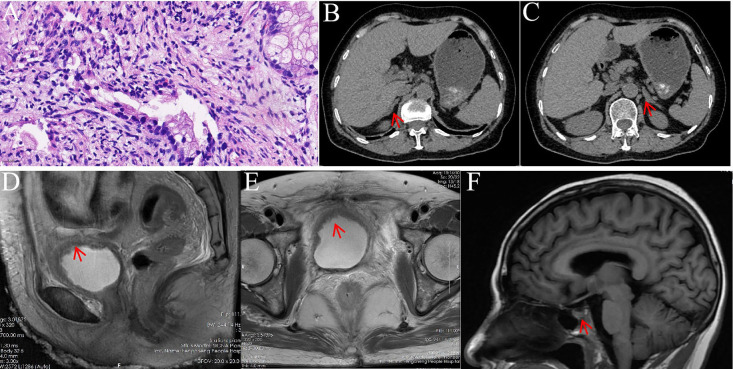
Pathological and imaging findings. (a) Depicts that Cervical biopsy showing GEA (HE, x400); (b, c) CT images of the right and left adrenal glands, respectively, demonstrating normal findings; (d, e) MRI images of the bladder in the sagittal and transverse planes, respectively, revealing diffuse and irregular wall thickening; (f) Sagittal MRI of the pituitary gland showing no abnormalities.
